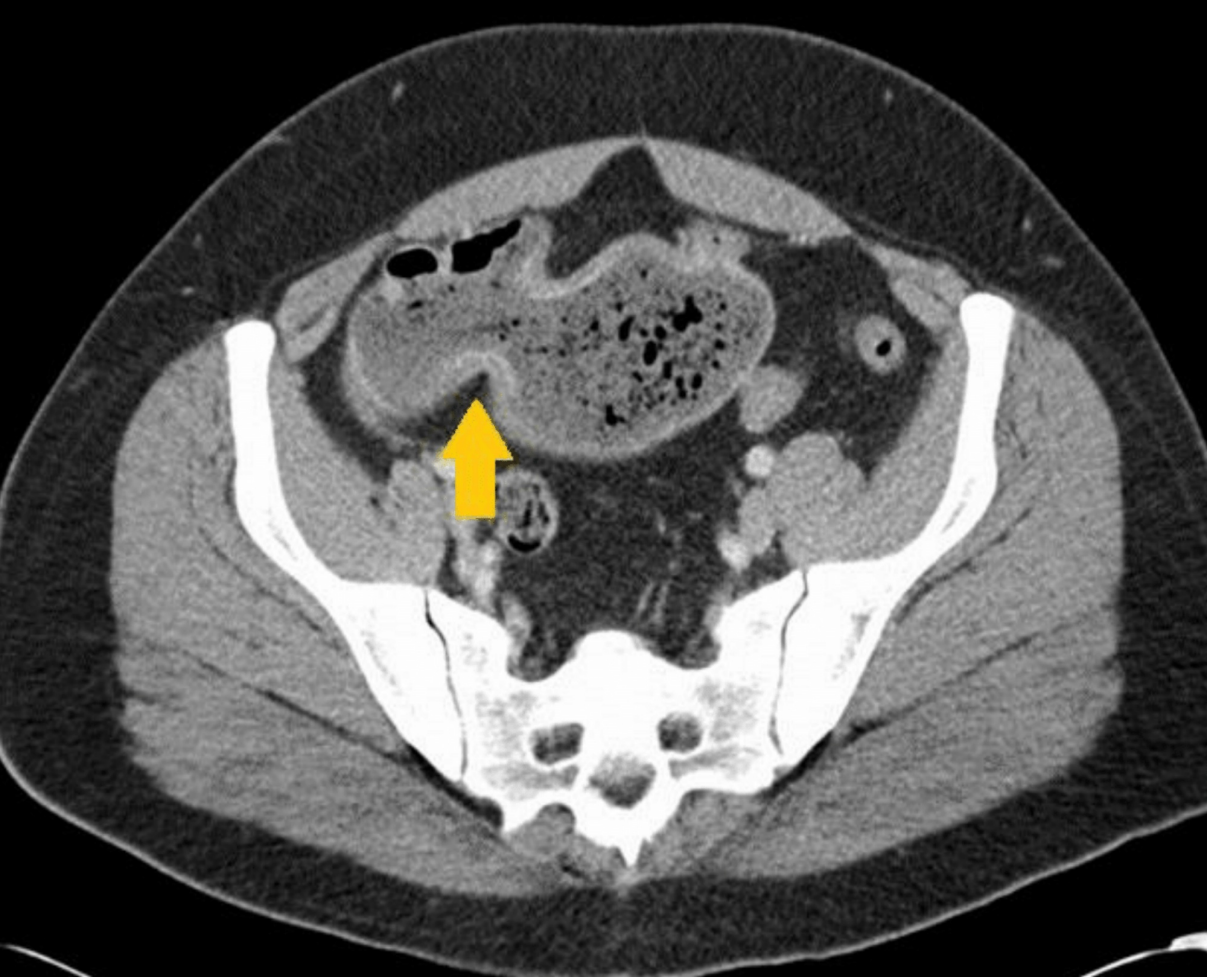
Contrast enhanced computed tomography (CT) abdomen/pelvis showing a segment of small bowel in the right lower quadrant with areas of stricture and dilation.
